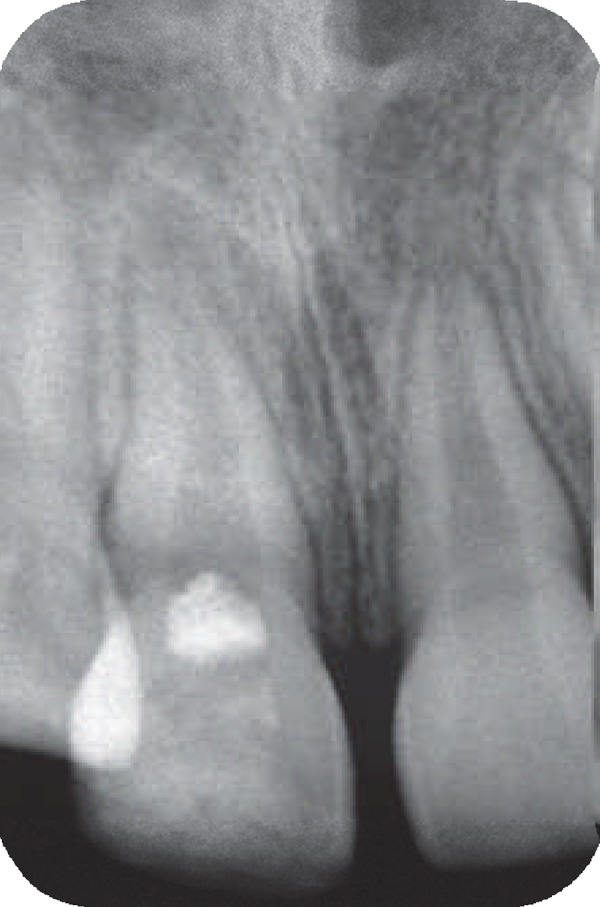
Radiographic image of the same tooth 6 months after treatment
